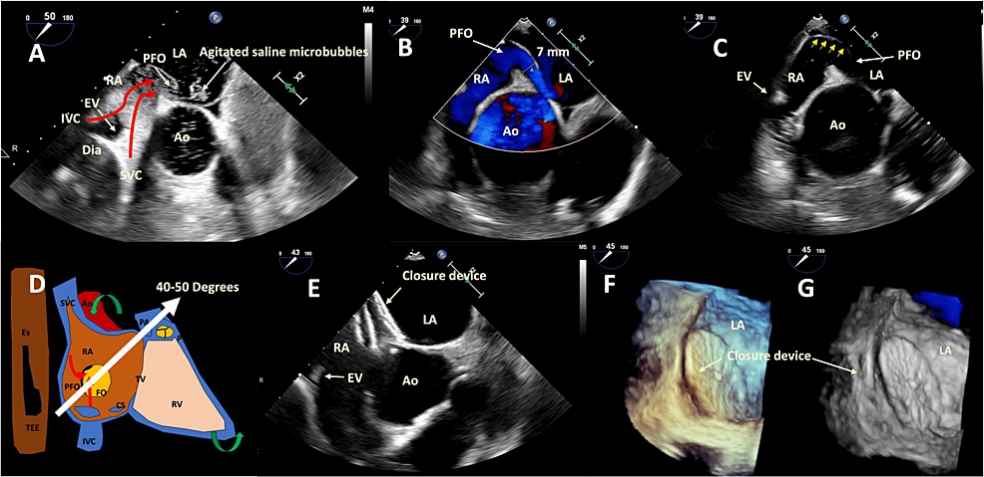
TEE images in modified mid-esophageal views depicting the flow-directed, right-to-left shunt through the PFO. (A) Initial TEE with agitated saline via an upper extremity peripheral vein, revealing a large PFO with a right-to-left shunt. This TEE was done about eight months before the images were obtained in (B) through (G). Note the marked increase in the ascending aorta size from (A) 3.5 cm × 3.5 cm to (B) and (C) 4.8 cm × 4.7 cm. The flow-directed, right-to-left shunt can be appreciated through the PFO on color flow Doppler. (C) Maneuvers that increase venous return through the right side of the heart can lead to a *spinnaker* effect toward the aneurysmal septum (yellow arrows), keeping it open and worsening the intracardiac shunt. (E) Occlusive device (Abbott Amplatzer 30 mm PFO Occluder, Abbott Park, IL, USA) after placement, (F) seen on three-dimensional TEE reconstruction with (G) color flow Doppler demonstrating the absence of a residual shunt. TEE, transesophageal echocardiogram; PFO, patent foramen ovale; RA, right atrium; LA, left atrium; Ao, aorta; SVC, superior vena cava; IVC, inferior vena cava; EV, Eustachian valve; PA, pulmonary artery; TV, tricuspid valve; Es, esophagus; CS, coronary sinus
